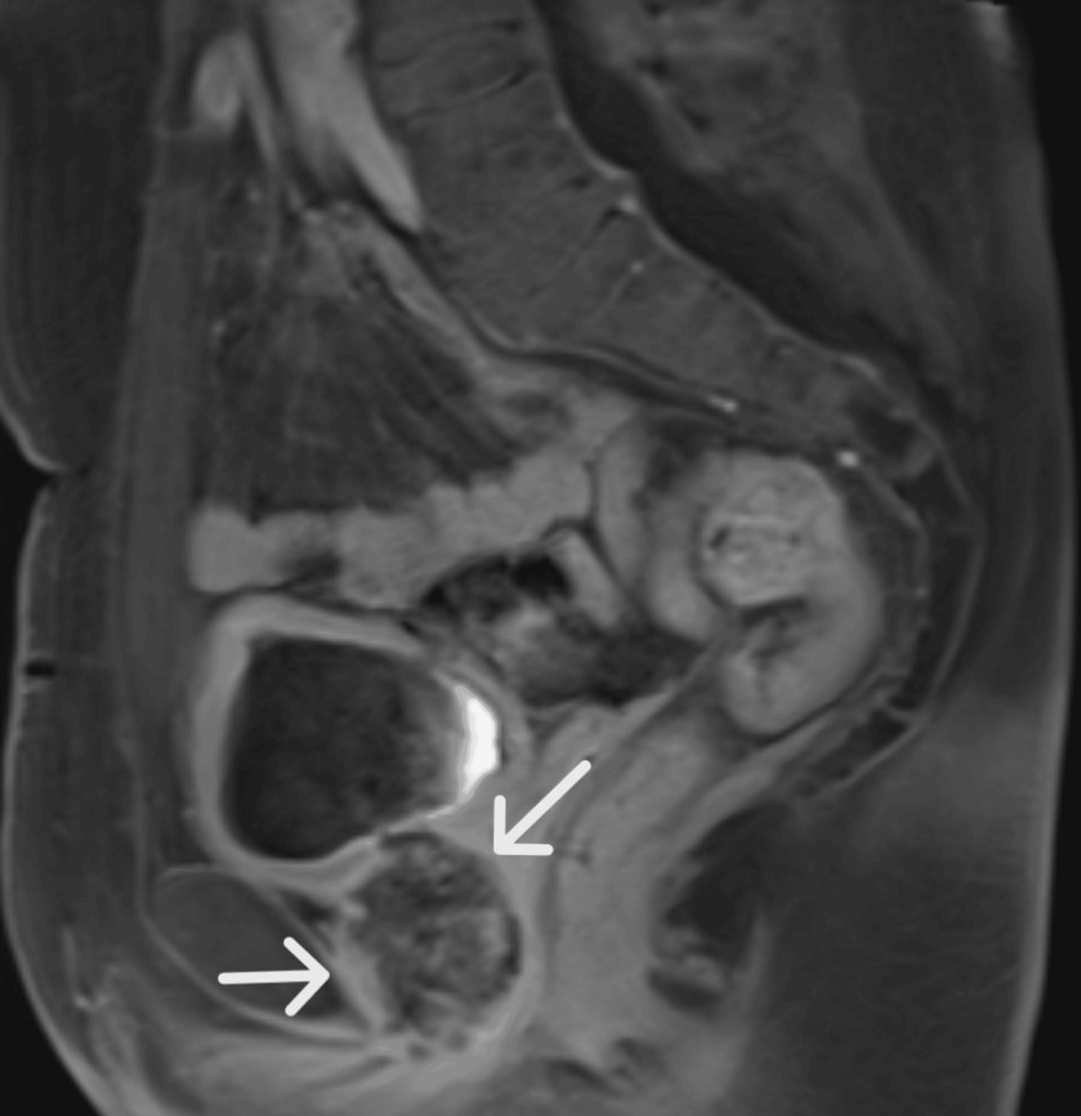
Sagittal T1-weighted postcontrast magnetic resonance image of a heterogeneously enhancing periurethral mass just beneath the urinary bladder, denoted by the white arrows
